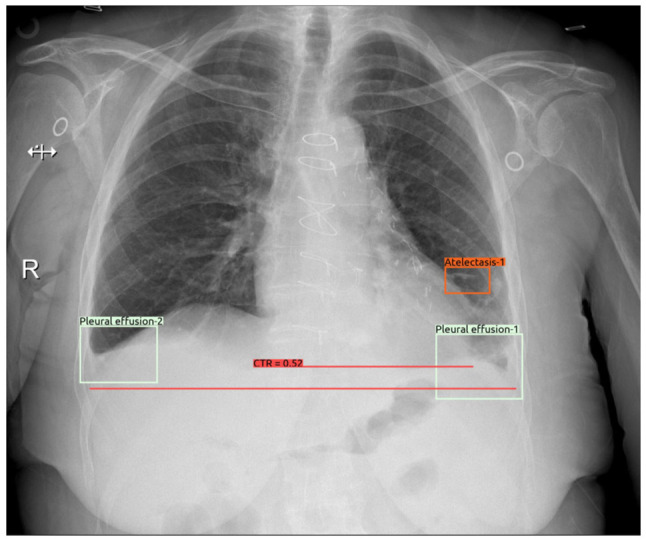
Bilateral pleural fluid, cardiomegaly and metallic suture and clip secondary to coronary artery bypass graft and surgical aortic valve replacement were determined in the chest X-ray.
